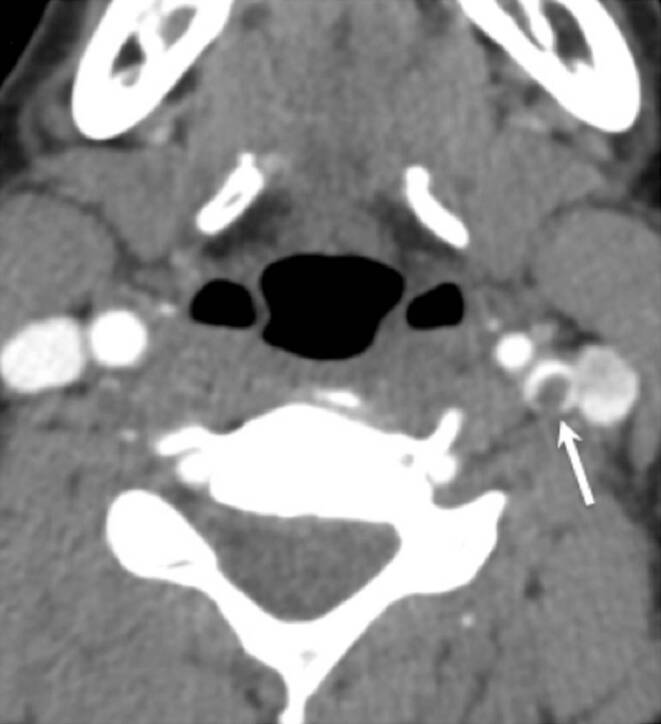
Cross sectional CT angiogram of the neck showing free floating thrombus in the proximal ICA resulting in severe stenosis of the ICA (*white arrow*)
